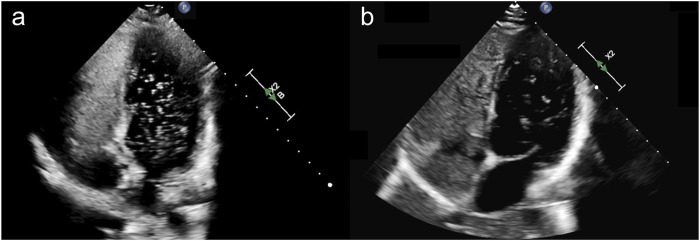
(A) Apical four-chamber view during transthoracic contrast echocardiogram with an agitated saline bubble study in index case (II-4) upon initial evaluation, demonstrating a delayed appearance of >100 bubbles in the left-sided cardiac chambers consistent with a Grade 3 intrapulmonary shunt. (B) A repeat study during 6-month follow-up showed delayed appearance of 30–100 bubbles consistent with a Grade 2 intrapulmonary shunt.
